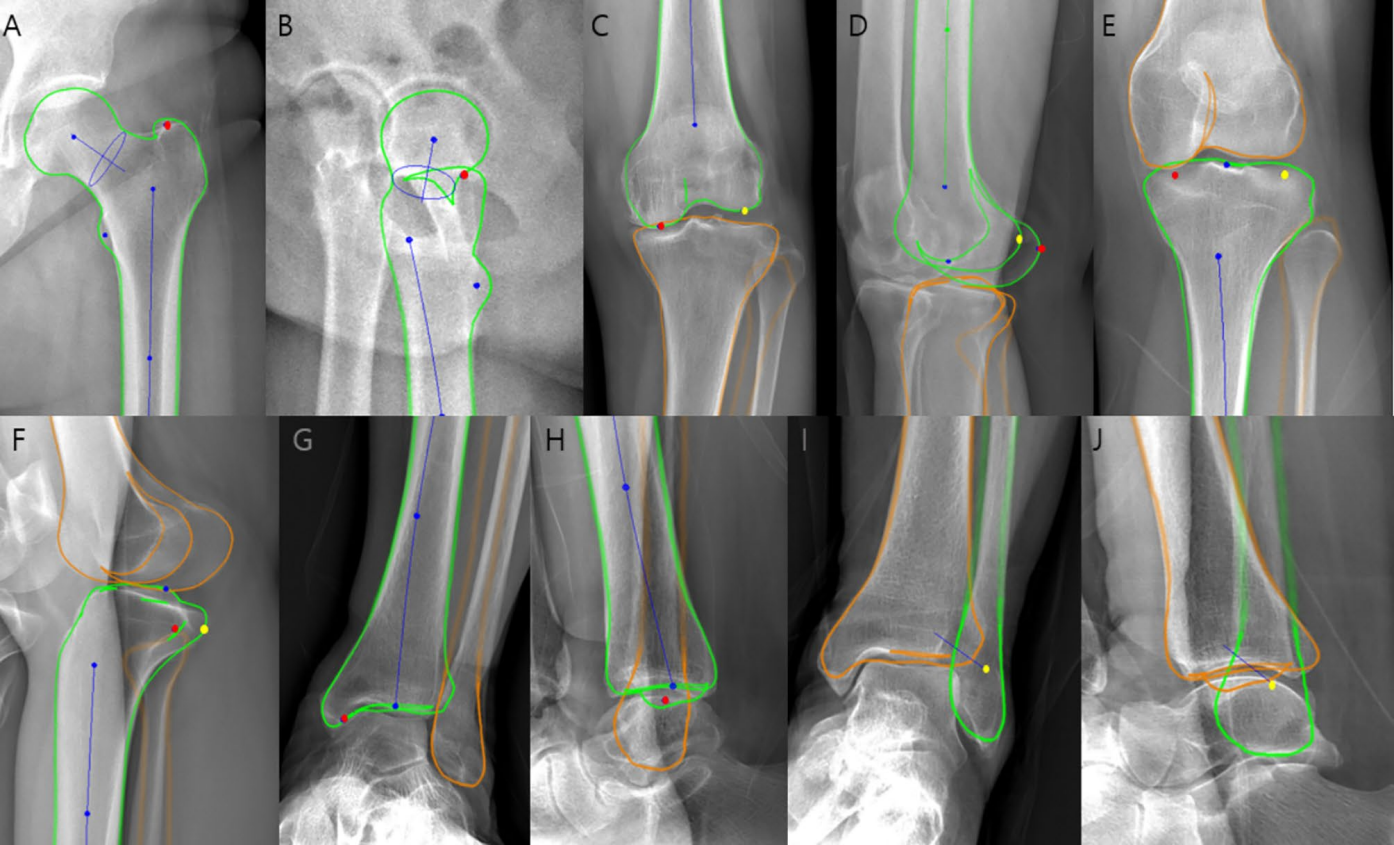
Three-dimensional model based on biplanar radiographs. (A–J) Computer model (green) is adapted semi-automatically to the osseous contours of the femur and tibia on anteroposterior (A, C, E, G, I) and lateral (B, D, F, H, J) radiographs. Three-dimensional models are built based on the fitting process, which is facilitated with standardized points on the osseous contour that can easily be adjusted by dragging and dropping. The key points that are crucial for torsion measurements are the greater trochanter at the hip (red point on A, B), the posterior contours of the femoral condyles (red points on C, D: medial condyle; yellow points on C, D: lateral condyle), the posterior contours of the medial and lateral aspects of the tibial head (red points on E, F: medial aspect; yellow points on E, F: lateral aspect), and the malleoli of the ankle joint (red points on G, H: medial malleoli; yellow points on I, J: lateral malleoli).
